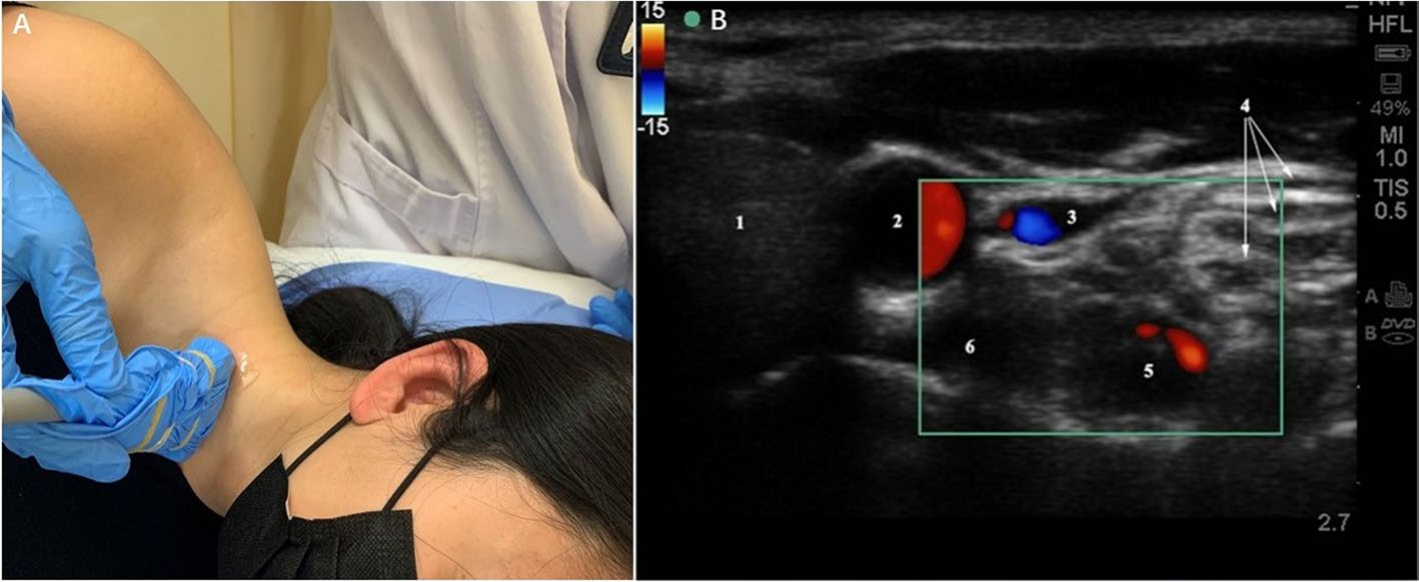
Ultrasonic exploration of stellate ganglion. A: Patient’s position and placement of ultrasound probe. B: Ultrasound image of the cervical structure during stellate ganglion block.1, thyroid; 2, common carotid artery; 3, internal jugular vein; 4, brachial plexus; 5, vertebral artery; 6, longus colli muscle
